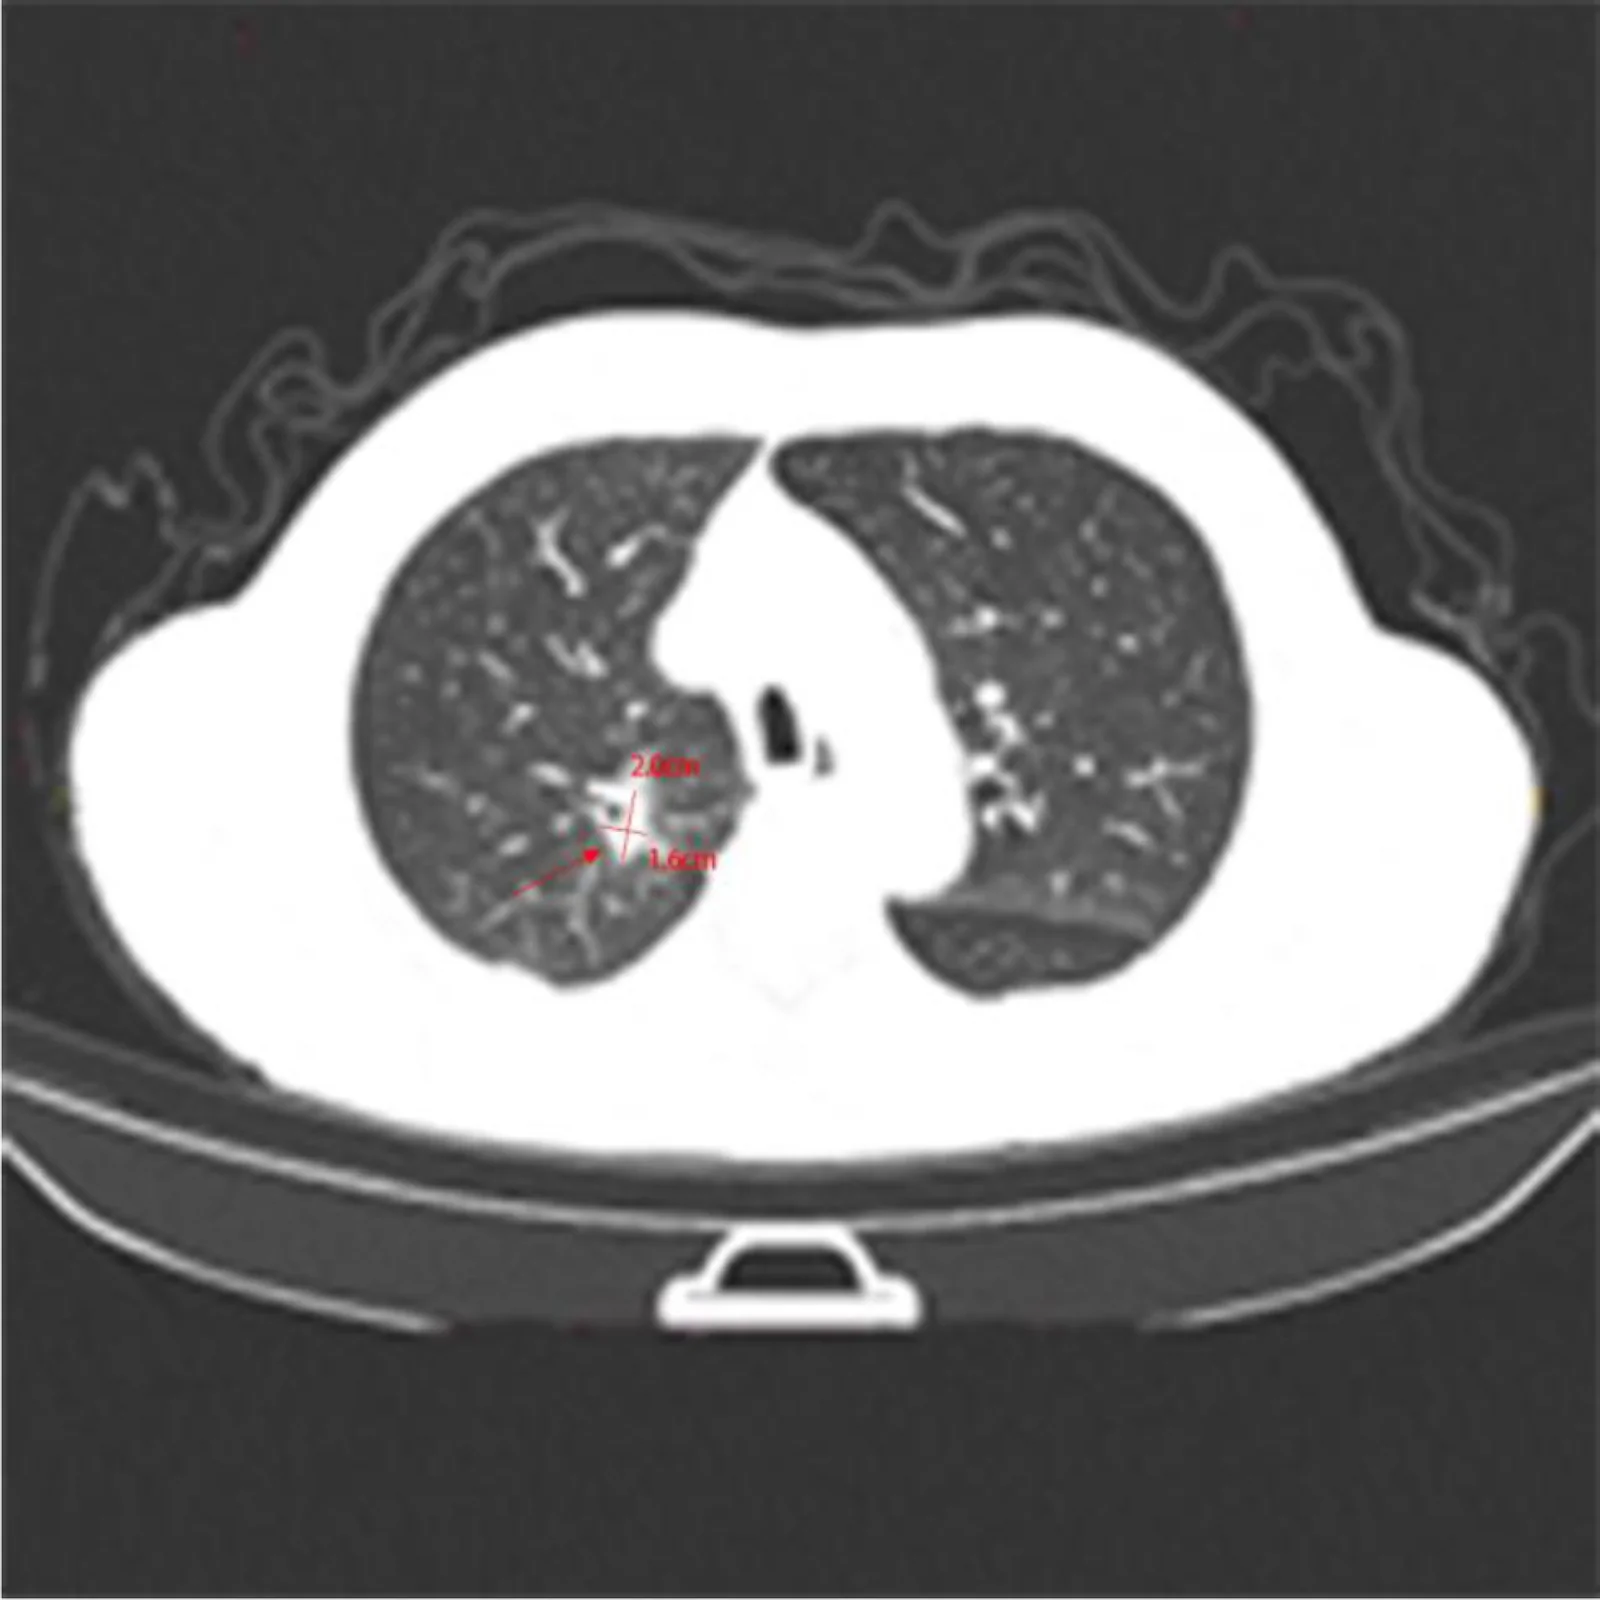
January 2021, lung window of CT scan showed a 1.6 × 2.0cm right pleural nodule. CT = computed tomography.
